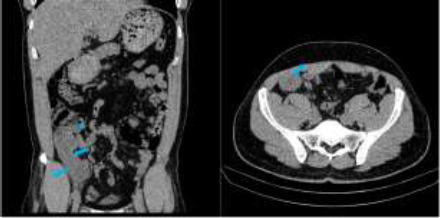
Non contrast coronal reformatted and axial CT scans of the abdomen and pelvis, demonstrating a large blind-ending tubular hypodense mass (long arrows), continuous with the caecum (short arrow) and consistent with a grossly dilated appendix
